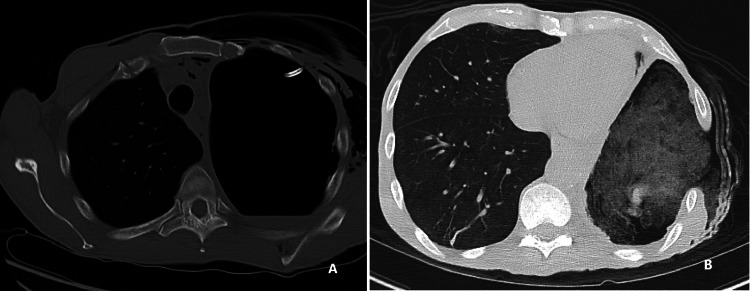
CT scan chest of the same patient above showing post left upper lobectomy. (A) Infected pleural space with multiple pleural adhesions and air-fluid level in the left lung base. Atelectasis in the remaining left lower lobe. Soft tissue emphysema of the left hemithorax. S/p chest tube for empyema drainage. (B) CT chest of the same patient five months later showing left upper lobectomy seen previously and interval creation of a left Claggett window. Packing material filling the left pleural space is associated with atelectasis of the left lower lobe. No collections identified in the left hemithorax. Images Courtesy by Dr. Ory Wiesel
